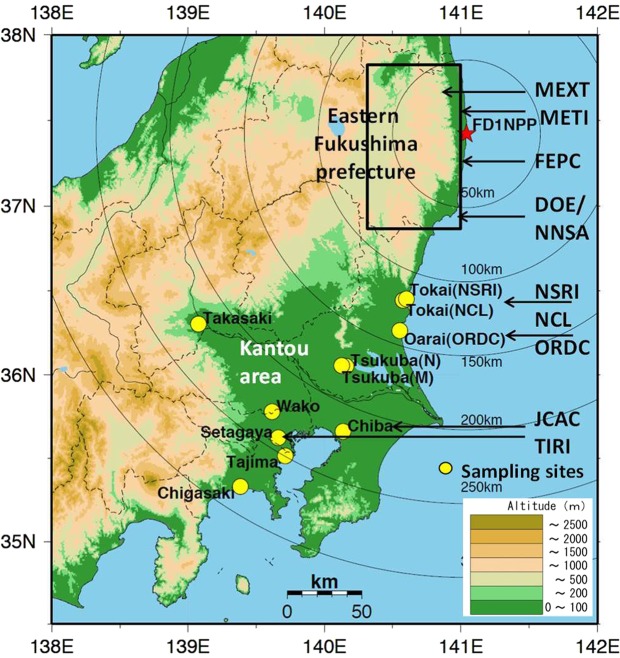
Map of the sampling sites or areas for atmospheric nuclides in the early period after the accident. Detailed information is listed in Table 1. In the Kantou area, atmospheric radionuclides were measured at 11 sites, and atmospheric ^137^Cs and ^131^I concentrations at NSRI, JCAC, and TIRI were analysed in detail in this study. The sampling sites of Tsukuba(N) and Tsukuba(M) are NIES and MRI in Table 1, respectively. The sampling area for the eastern Fukushima prefecture is also shown, and each sampling point by MEXT, FEPC, DOE/NNSA, and METI is shown in Figs 6 and S5, respectively. The mapping was made by using the Generic Mapping Tools (GMT)^50^ and the topography data of USGS EROS Archive - Digital Elevation - Global 30 Arc-Second Elevation (GTOPO30)^51^.
